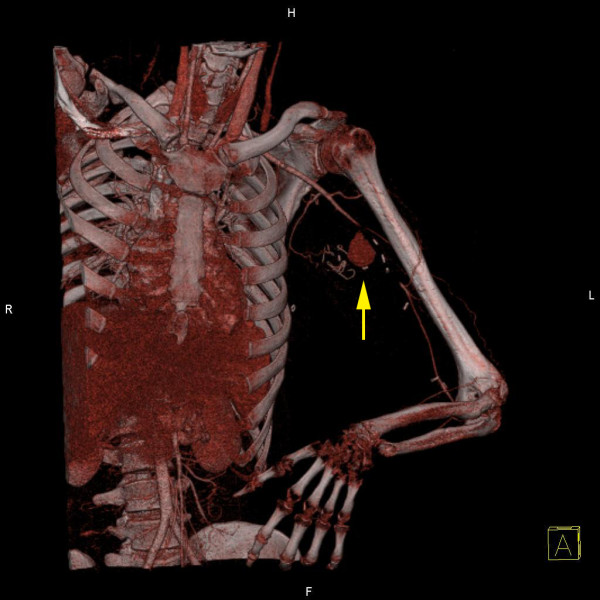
Preoperative three-dimensionally reconstructed angio CT scan. Three-dimensionally reconstructed angio CT scan. A pseudoaneurysm in the left brachial artery was noted.
